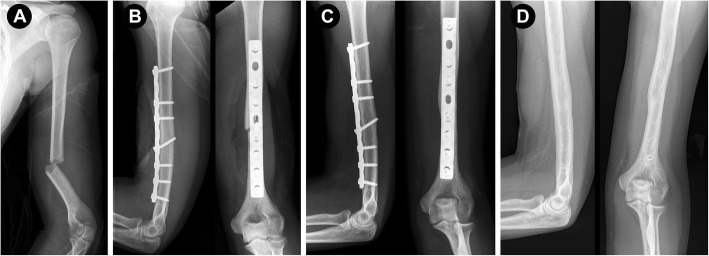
a–d. An 18-year-old female patient with an OTA type 12-A3 humeral fracture (a). Postoperative radiographs after open reduction and internal fixation (ORIF) utilizing an anterior limited contact dynamic compression plate (LCDCP) (b). Fracture healing in the same patient six months after ORIF. Although we aimed for absolute stability and primary fracture healing, callus formation, a sign of secondary fracture healing, was observed. c Radiographs one year after the index procedure and implant removal (d)
